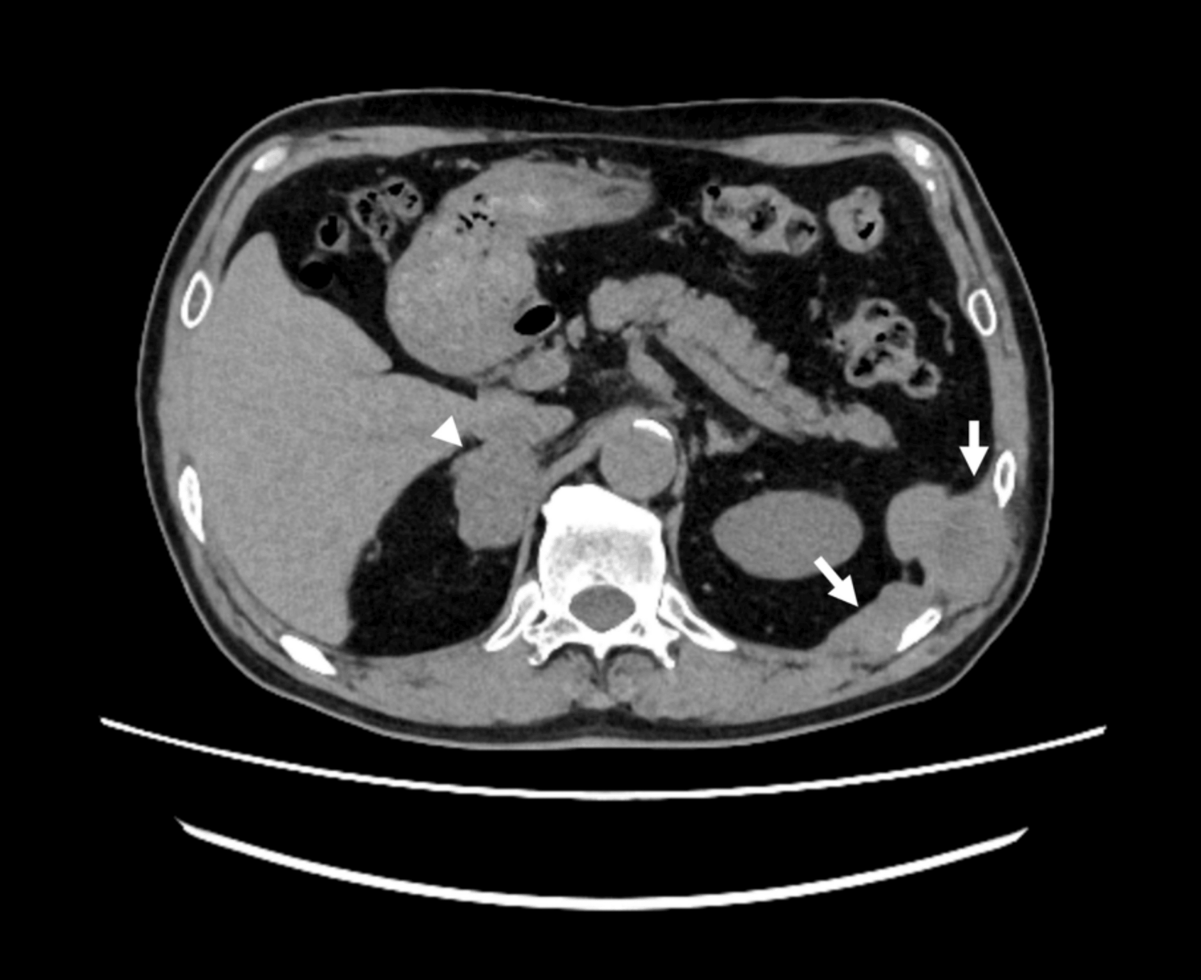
Abdominal CT image after chemotherapy The multiple pleural lesions exhibited increased size and extended specifically between the 10th and 11th intercostal spaces, resulting in abdominal discomfort on the left side (arrows). Additionally, metastasis to the right adrenal gland became evident on imaging (arrowhead), indicating further progression of the disease.
